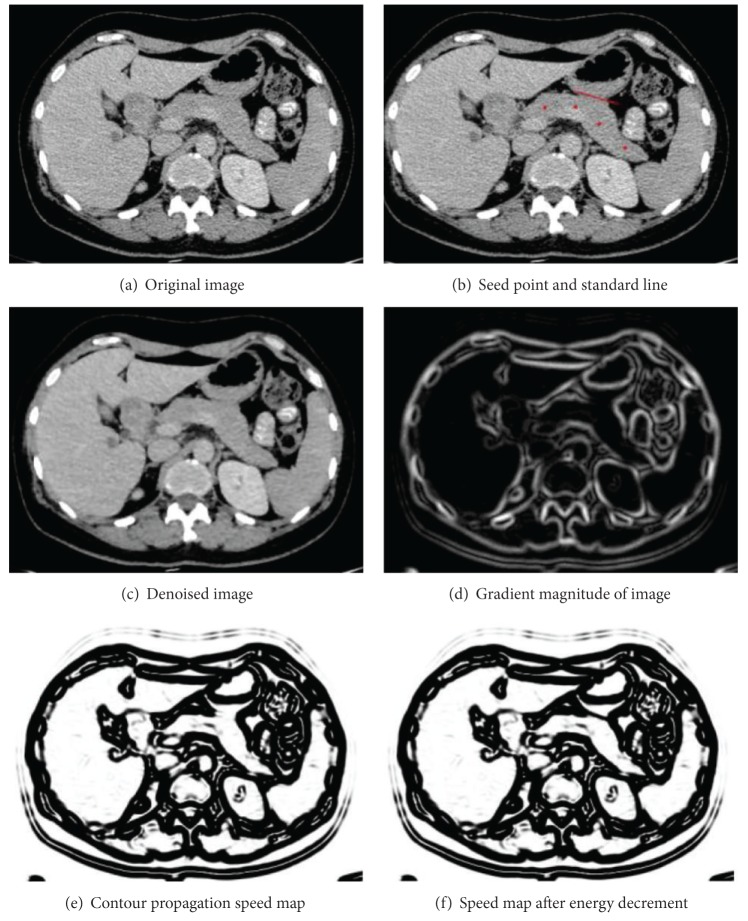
Denoising of image and feature maps of CT image. (a) Is original CT image, (b) is selection of seed points and standard line definition, (c) is denoised image processed by anisotropic diffusion filter, (d) is gradient magnitude map, (e) is edge feature map, which is employed in fast-marching level method, and (f) is energy map generated by decrement energy of speed map.
